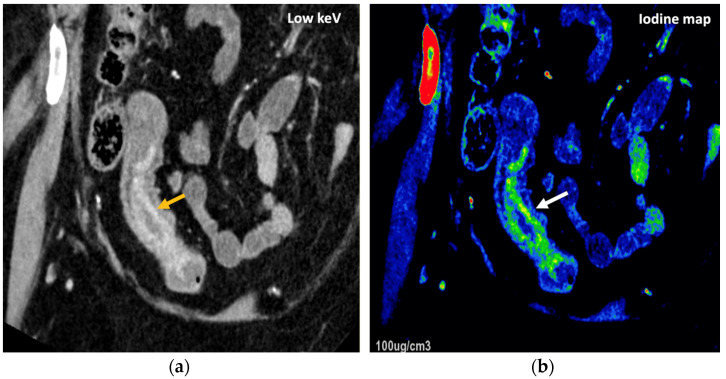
DECT offers definite advantages over single-energy CT to diagnose and assess disease severity of inflammatory bowel disease, particularly Crohn’s disease. A low-energy-level (45 keV) monochromatic reconstruction (a) and a color-coded iodine map (b) evidence increased enhancement and higher iodine concentrations, respectively, in the terminal ileum (orange and white arrows), which correlate with active inflammation in a patient with Crohn’s disease.
